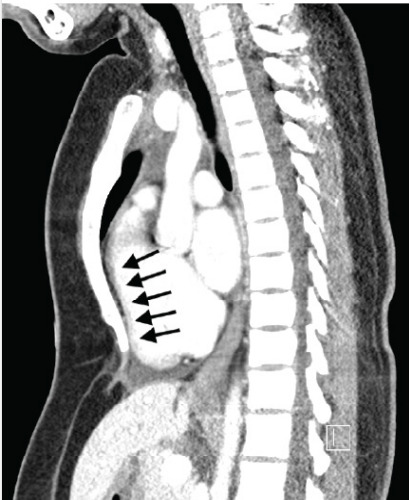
Contrast-enhanced chest computed tomography (sagittal plane) demonstrating right ventricular compression from severe pectus excavatum (black arrows).
